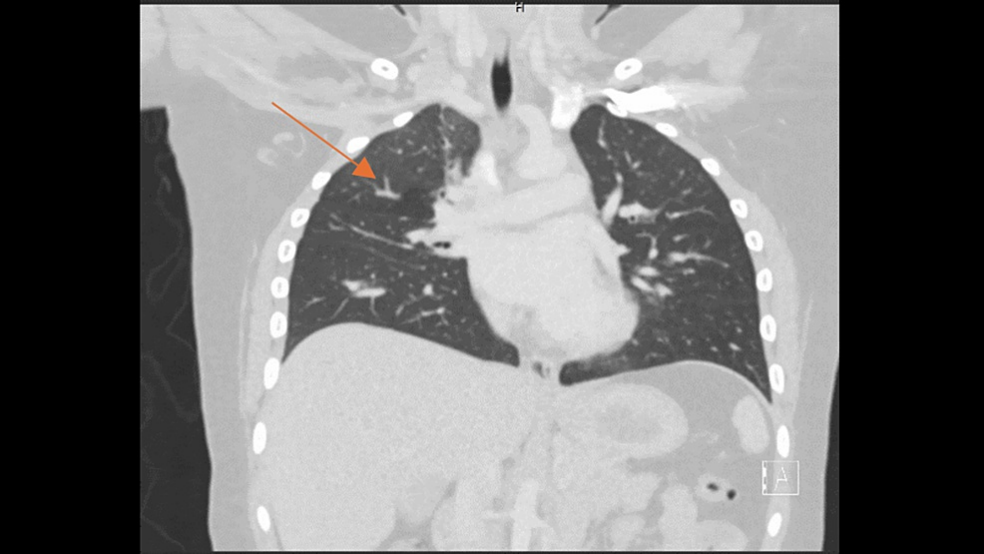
Repeat CT scan seven months later showing the resolution of the previously seen inhomogeneous opacification/cysts (frontal view). The arrow pointing to the resolution of the previously seen inhomogeneous opacification/cavity.
